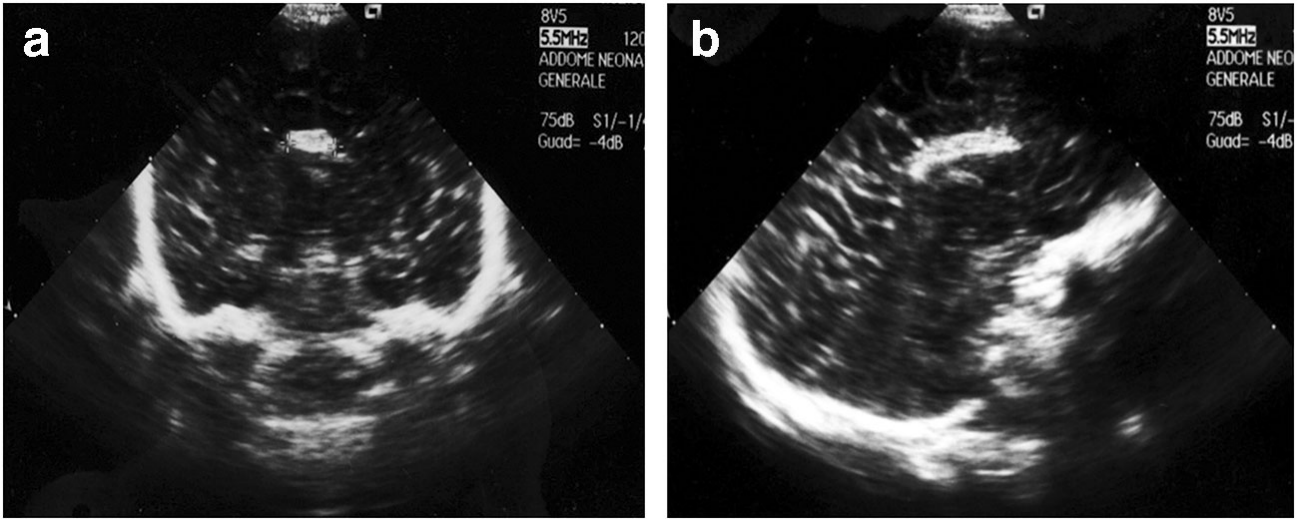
Cranial ultrasound in coronal and sagittal views: agenesis of the corpus callosum and a callosal lipoma, appearing as an interhemispheric midline echogenic mass [7]
